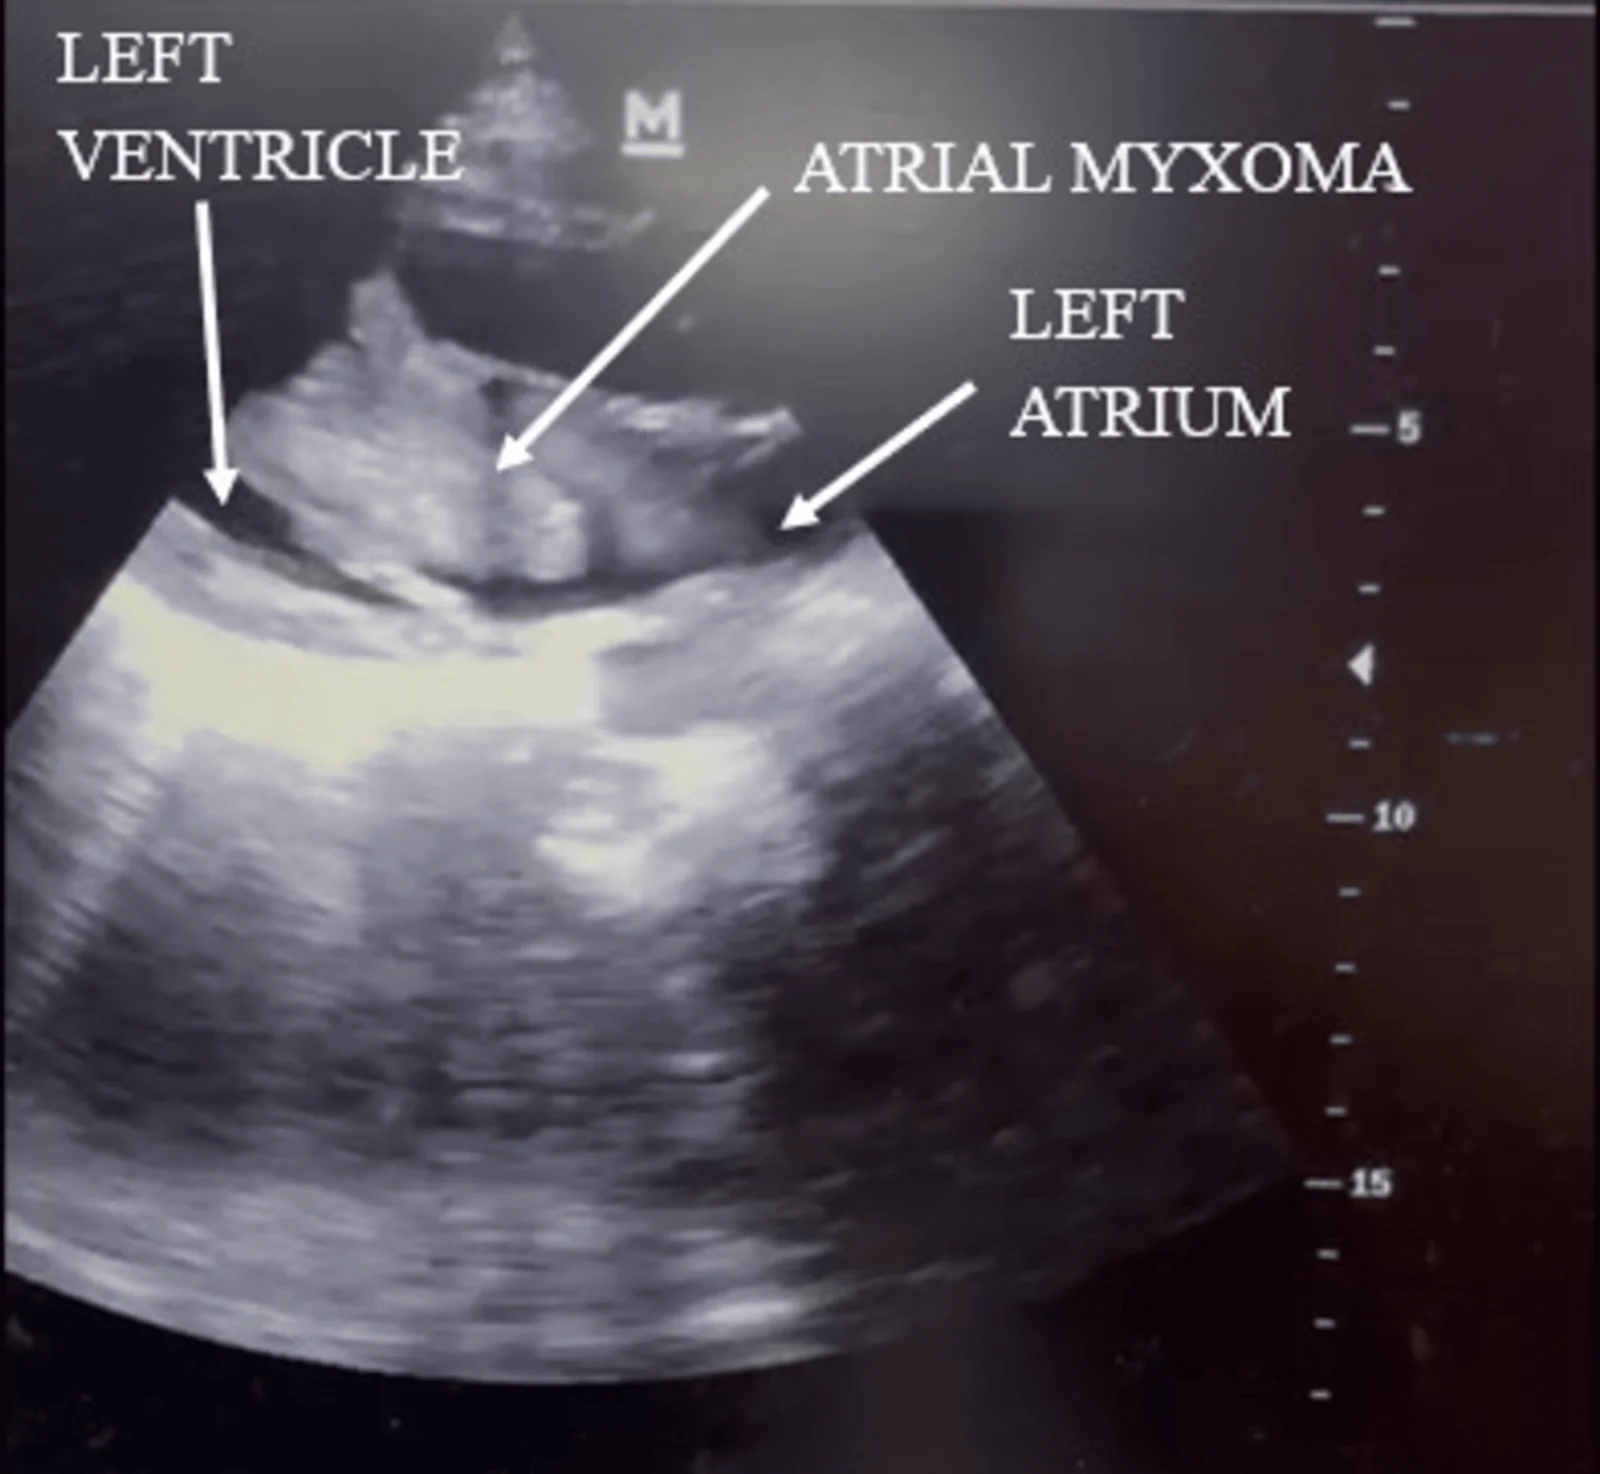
Echocardiography, which revealed an 82*40 mm large echogenic heterogeneous mass in left atrium attached to the interatrial septum, which was mobile and protruding into the left ventricle. The appearance was suggestive of a left atrial myxoma.
